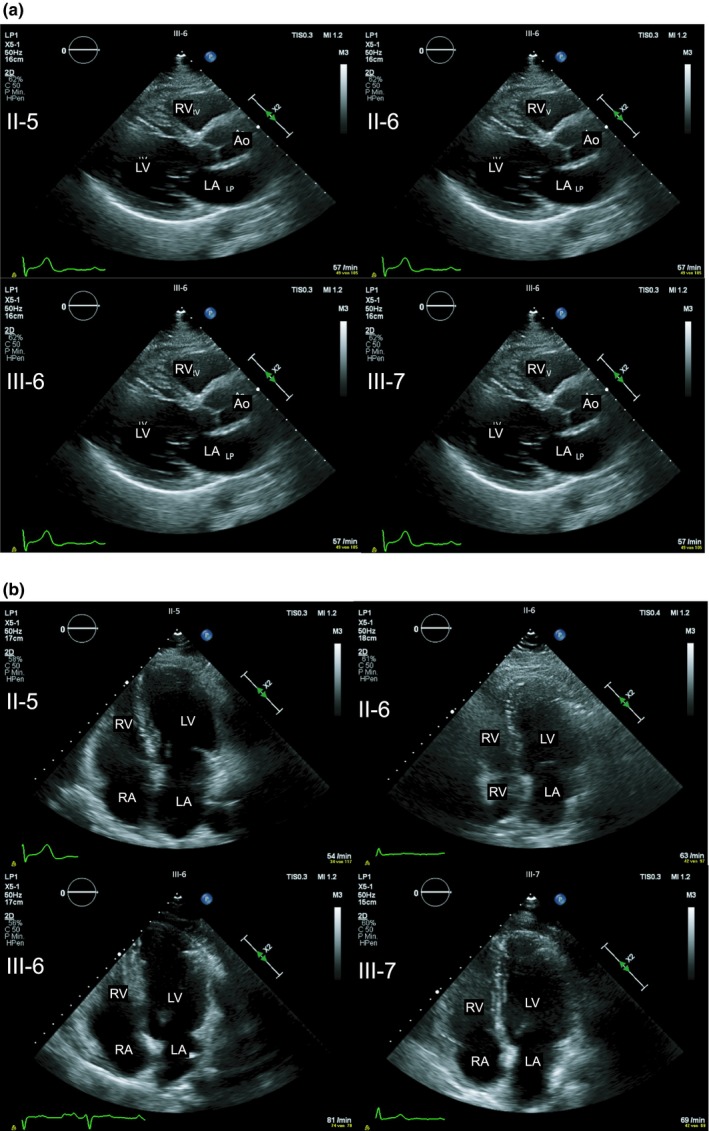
Echocardiography of family members (II‐5, II‐6, III‐6 and III‐7) reveals no further cases of hypertrophic cardiomyopathy. (a) Parasternal long axis and (b) four‐chamber view. LA = left atrium, LV = left ventricle, RA = right atrium, RV = right ventricle, Ao = aorta. For echocardiographic details s. Table 2
